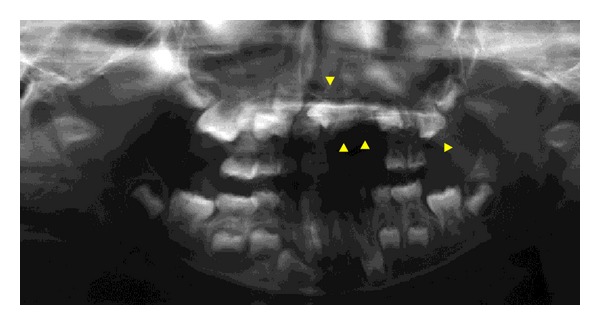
Panoramic radiograph at initial visit (case 1). Several permanent maxillary teeth are malformed and hypocalcified (arrowheads).
